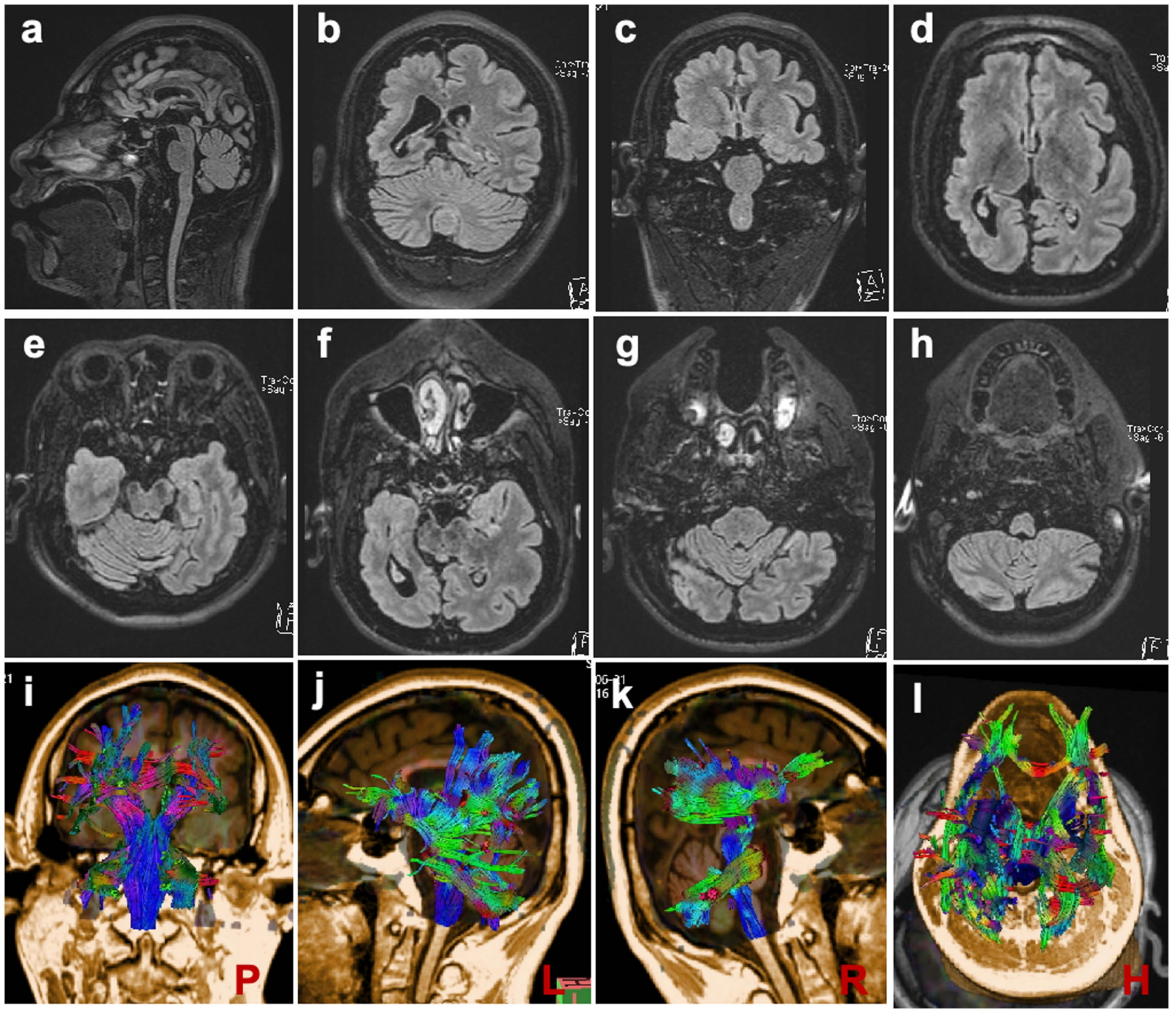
Brain MRI Scans (A) Scans demonstrating reduced brain volume and thinning of the corpus callosum. (B–D, F) Scans depicting widened sulci, reduced gyri, and dysplasia of the right cerebral hemisphere with bilaterally unequal ventricles. (E–H) Scans showing slight atrophy of the brain stem and cerebellum. (I–L) DTI series are presented at posterior, left, right, and horizontal angles, respectively.
